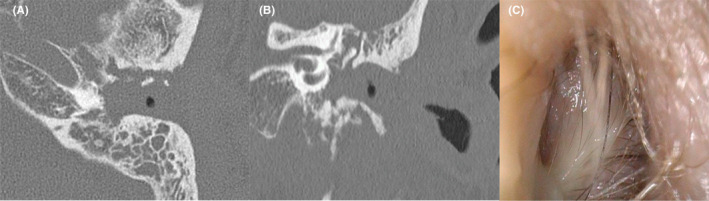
Computed tomography of the left temporal bone (A: Axial, B: Coronal). (C) Endoscopic findings of the external auditory canal
